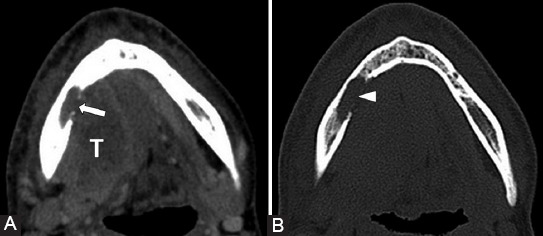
Squamous cell carcinoma (T) of the tongue base and oral floor. Soft-tissue (A) and bone window (B) axial computed tomography images demonstrate tumor invasion in the mandibular body on the right (arrow) with bone erosion into the alveolar canal (arrowhead), suggesting PNS along V3.
